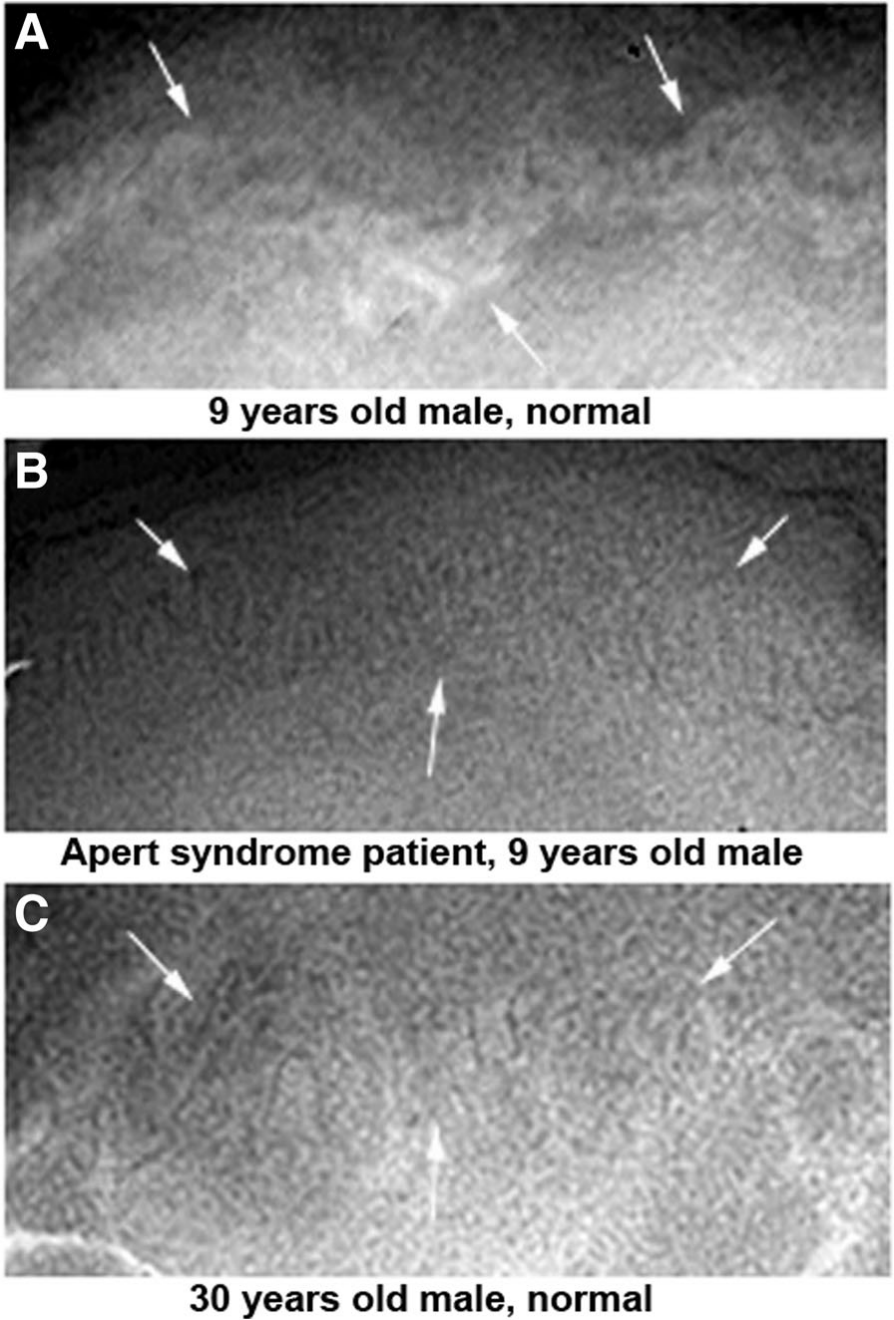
Coronal sutures in PA X-ray views. a A 9-year-old normal male with still immature coronal suture (arrows). b An Apert syndrome patient, a 9-year-old male, with tightly interdigitated coronal suture (arrows) similar to that of a 30-year-old normal male (c)
